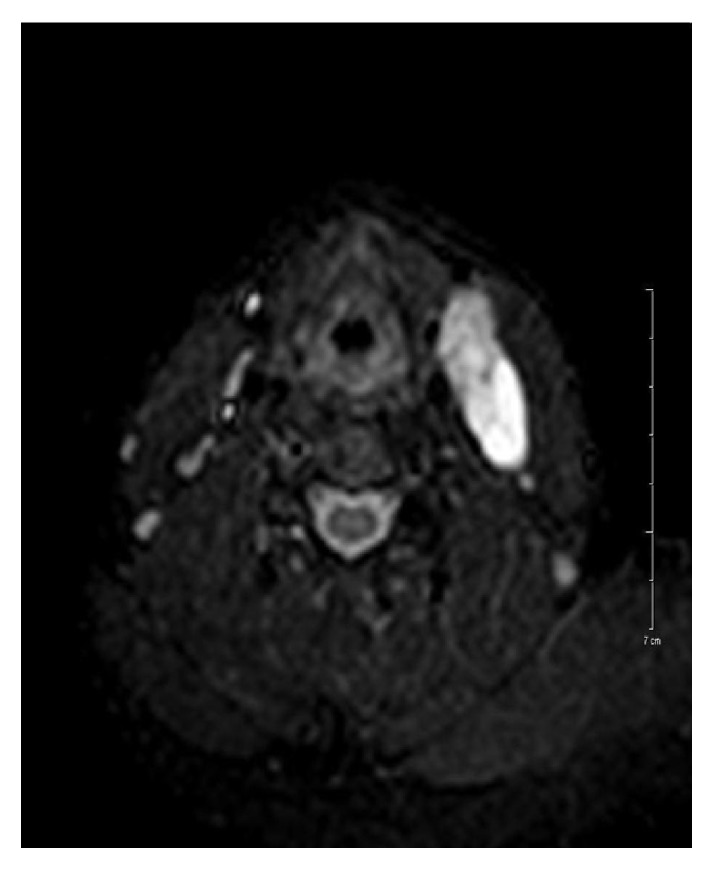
Contrast enhanced, axial sliced MR imaging of the cystic lesion.
